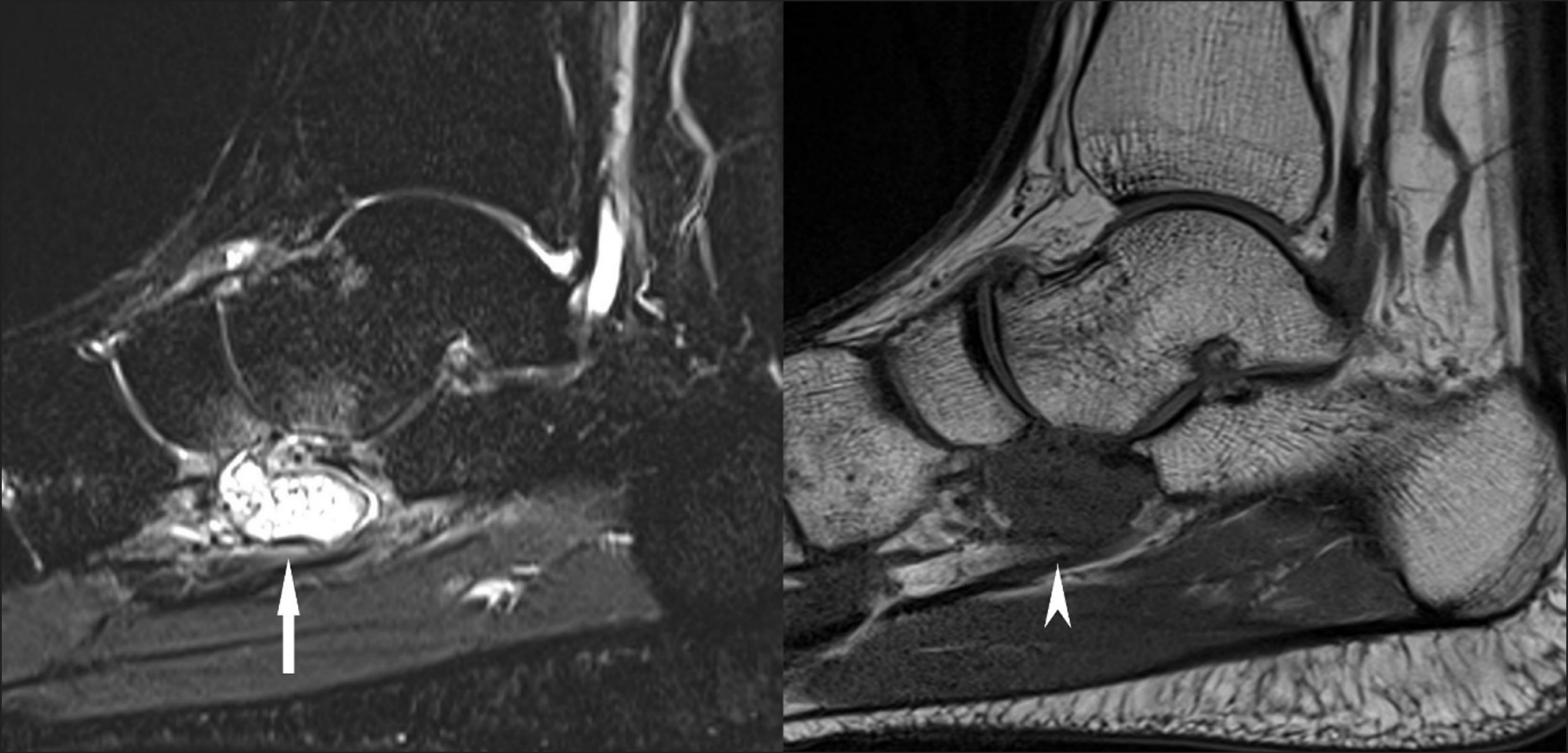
To clarify the findings on MRI, an additional computed tomography (CT) examination was performed. This demonstrated a 23 × 15 × 12 mm ossific density medial to the inferior margin of the cuboid and anterior to the inferior margin of the calcaneus (Figure 2, arrows) consistent with a very rare accessory ossicle called the os cuboideum secundarium.
